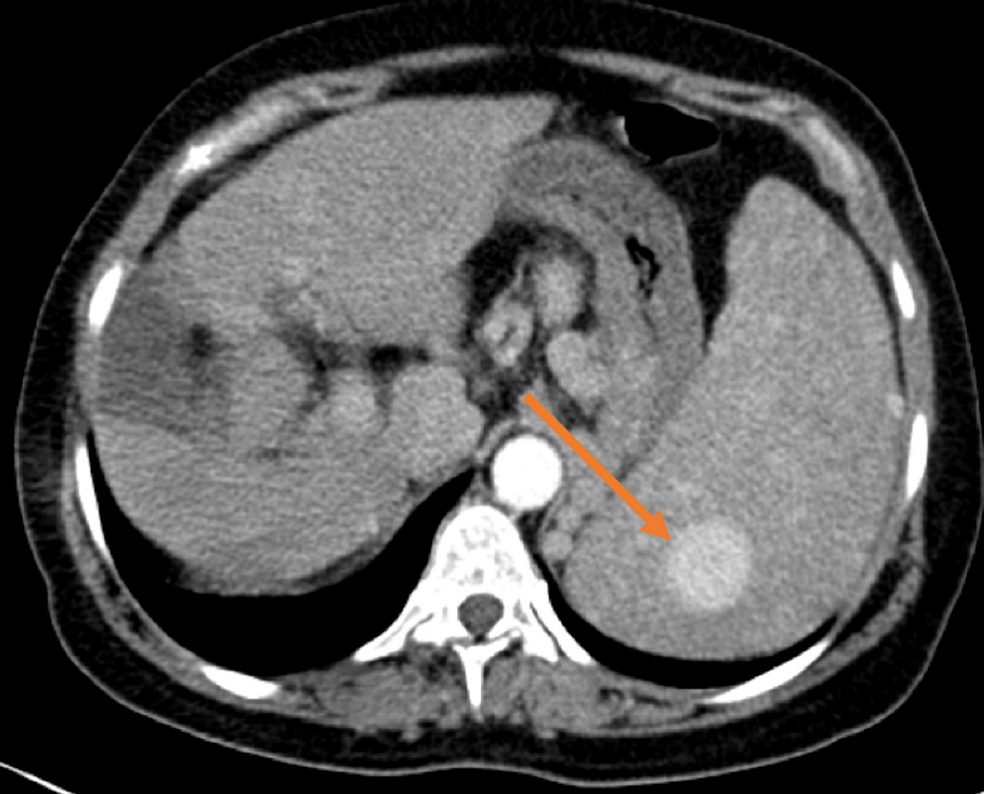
Axial view of abdominal CT scan (arterial phase) The image showed multiple nodular lesions corresponding to hemangiomatosis of the spleen; we also noticed the modifications of the liver contours CT: computed tomography
